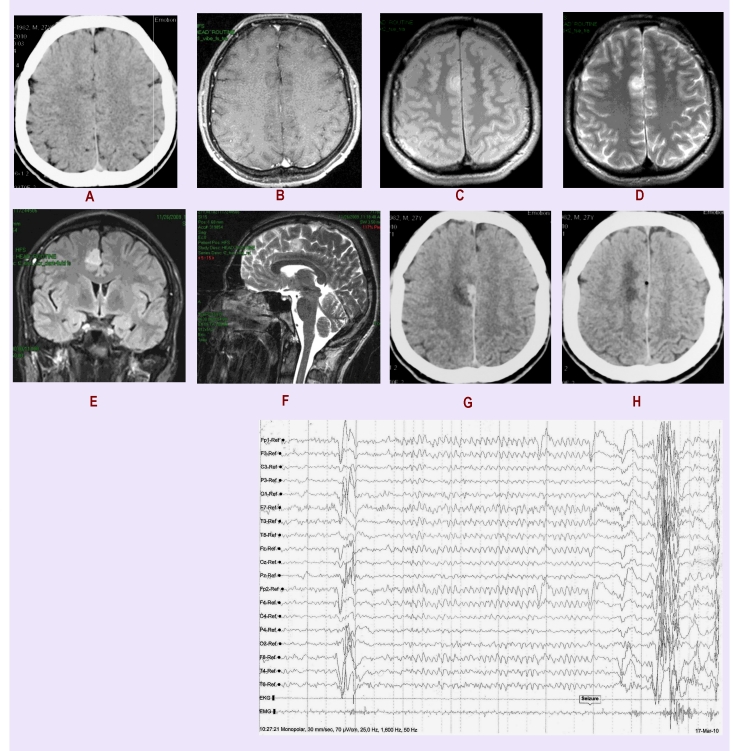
A. Preoperatory native CT scan: minimal hypodense frontal right paramedian lesion, B. T_1_ cerebral MRI small hyperintense parenchymal  homogeneous  lesion 16/22/15mm, well delimited, involving gyrus cinguli, without perilesional edema and mass effect, C hyperintense lesion on T_2_ MR sequences, non–enhancing after Gadolinium, D coronal lesion T_1_ MR sequences, E sagital lesion T_2_ MR sequences, G and H Postoperatory CT scan, I EEG recording: medio–frontal origin and rare interictal epileptiforme elements
